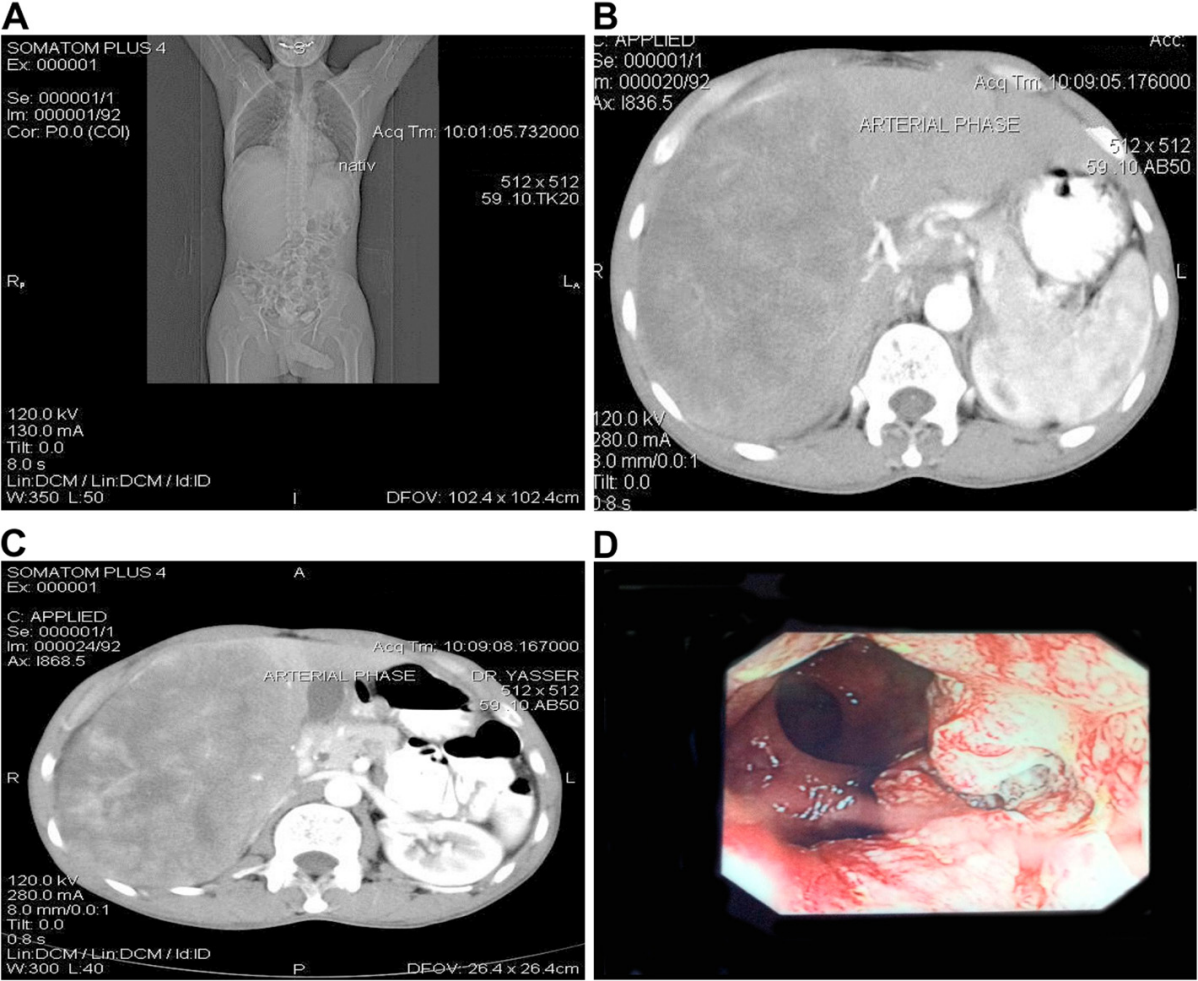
Imaging of the liver and rectal masses. (A) Scout film showing the large liver mass reaching down to the pelvis. (B) Computed tomography (CT) scan in the arterial phase showing the large liver mass involving the right hemi-liver, in close proximity to the main hepatic artery and involving the right hepatic artery. (C) CT scan in the venous phase showing the large liver mass compressing the right renal vein. (D) Colonoscopic image showing the mass lesion in the rectum.
